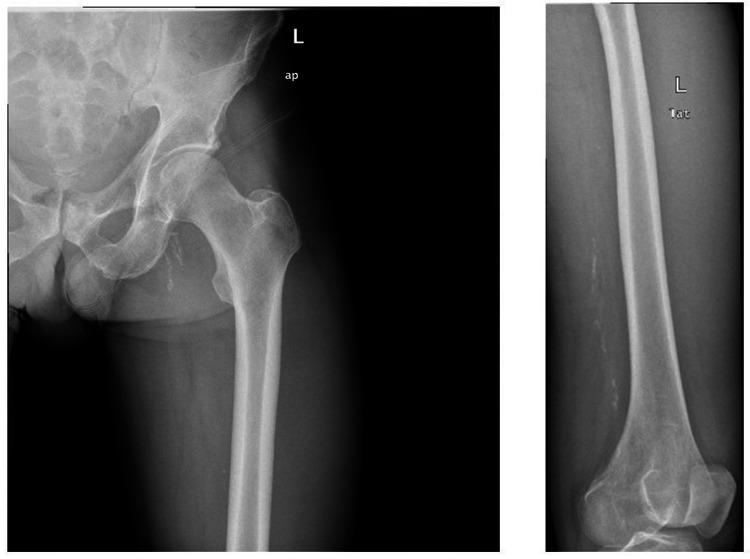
Hip and pelvic X-ray examination with no bone lesions.
